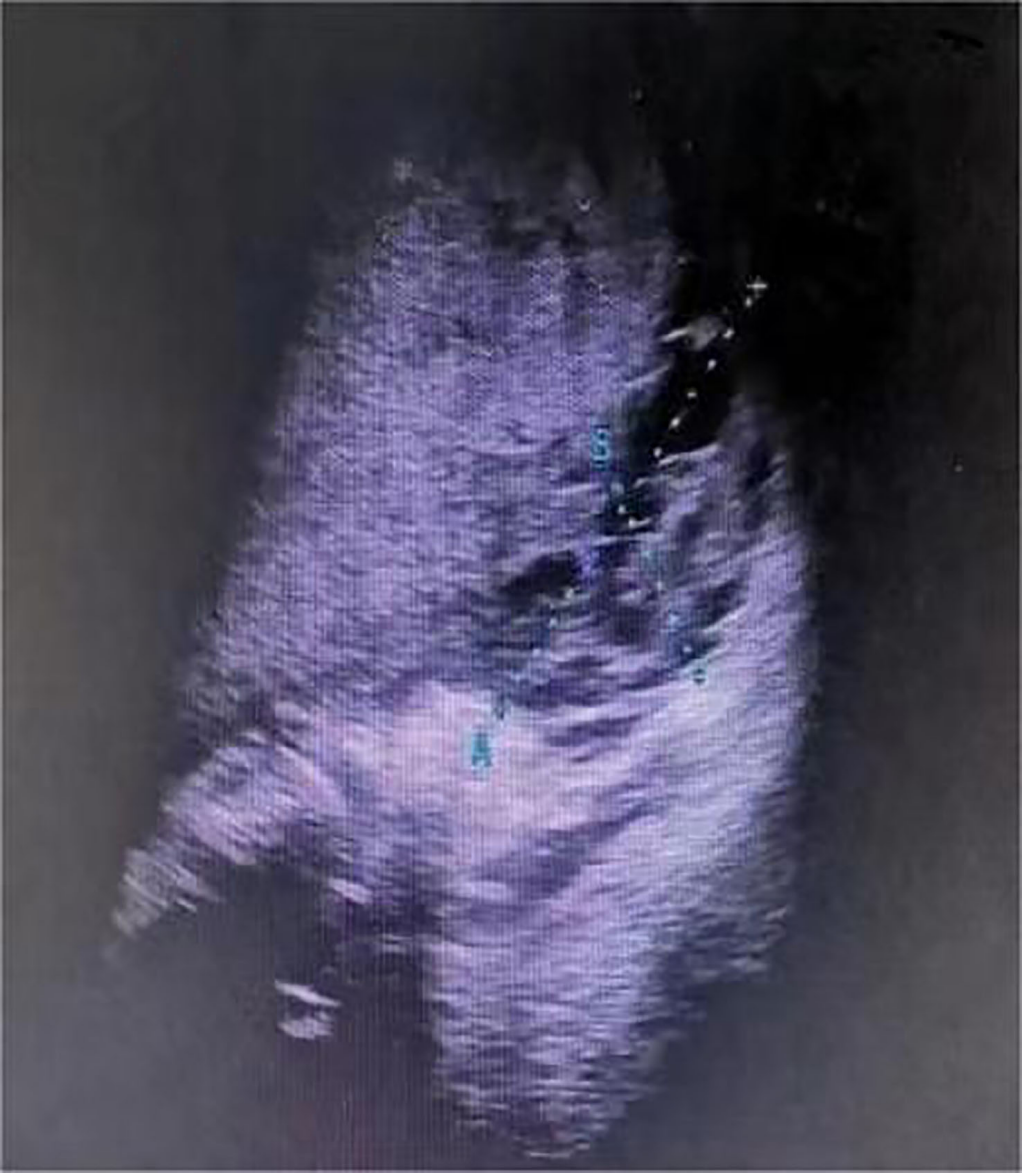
Obstetric ultrasound of the patient before cesarean section.
